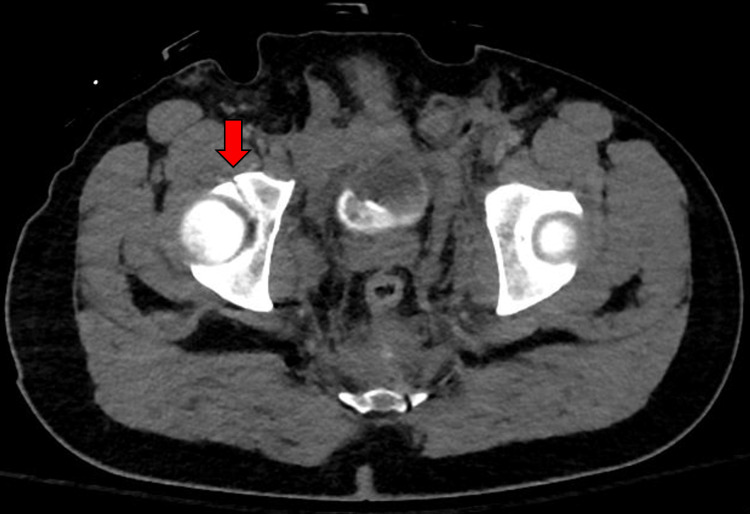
CT abdomen/pelvis demonstrating nondisplaced fractures of the right acetabulum (red arrow). CT: computed tomography
